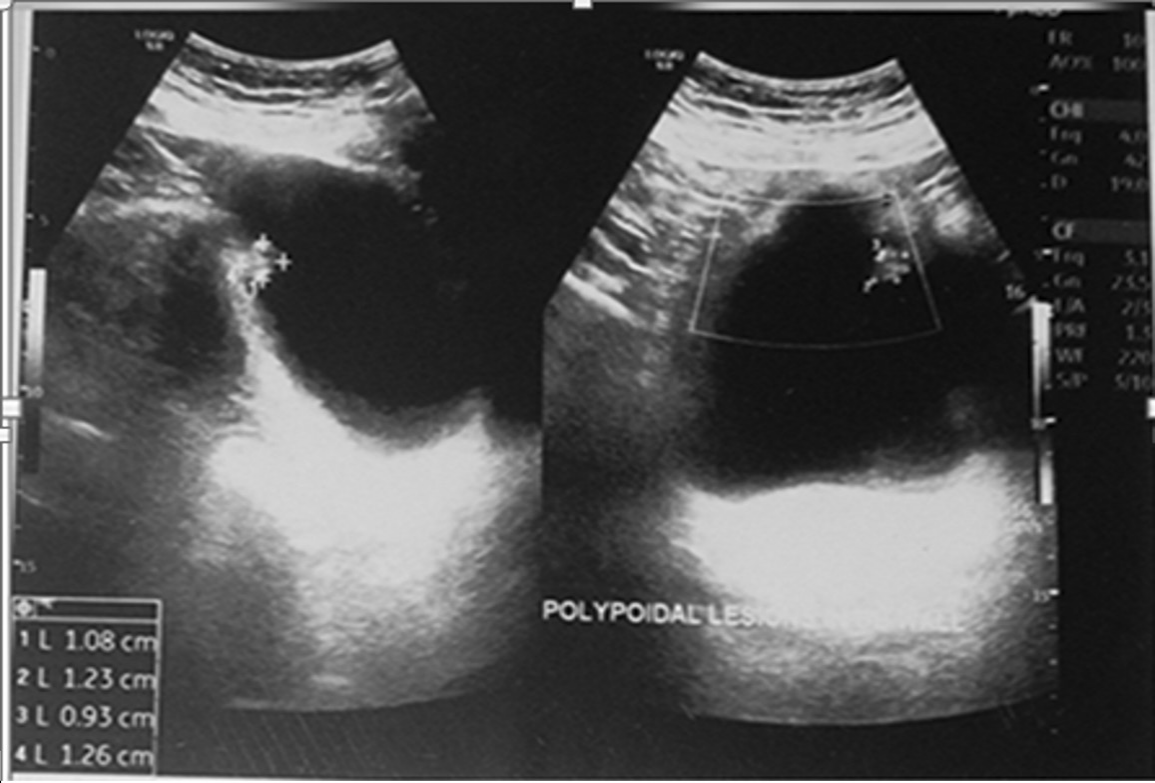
Transabdominal pelvic ultrasound showing urinary bladder, debris floating in urine with thickened urinary bladder wall (signs of cystitis) with a polypoidal lesion in the posterior wall of the urinary bladder (boundary marked with +).
